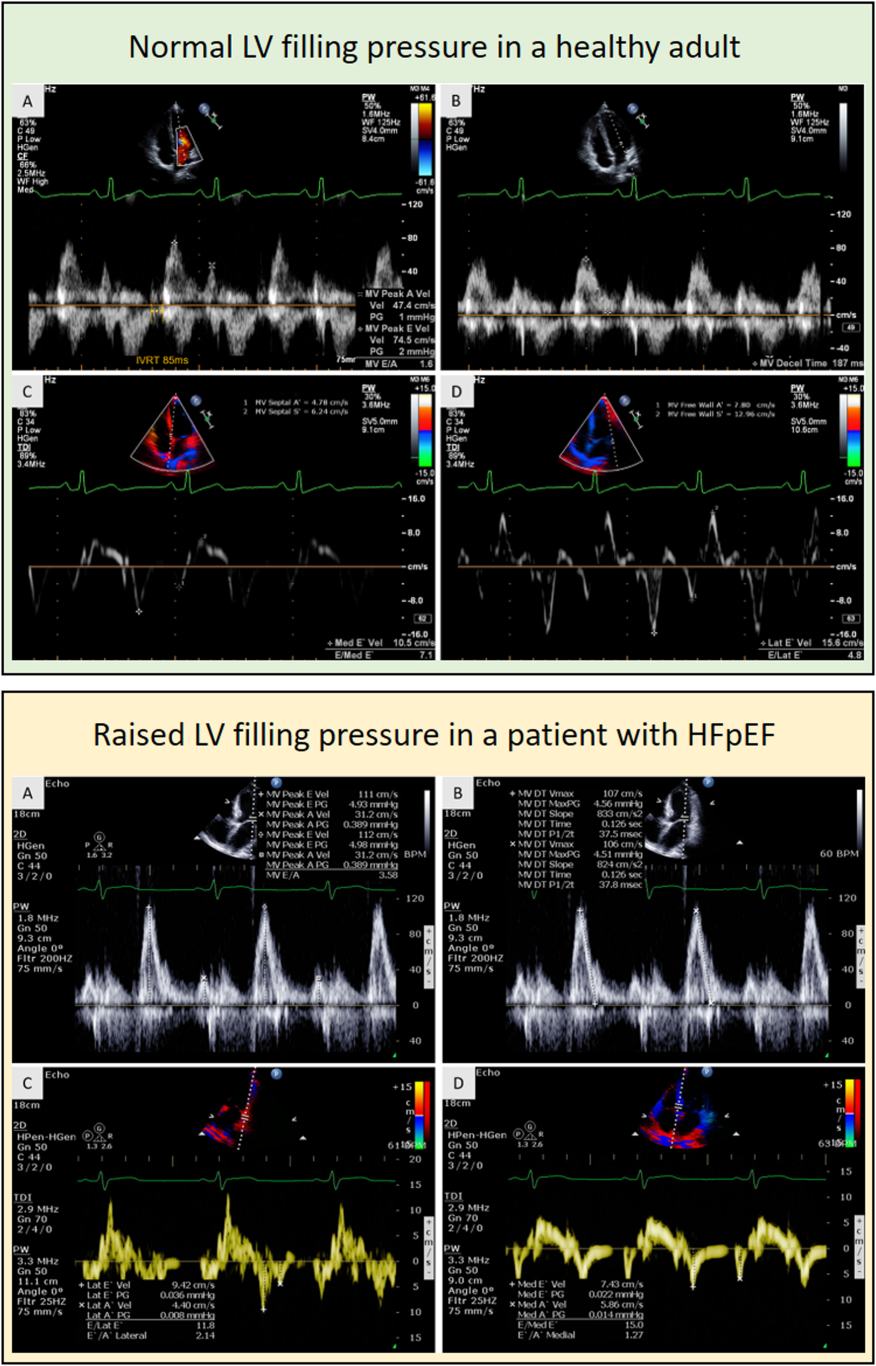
Case examples of Doppler‐based methods for left ventricular filling pressure (LVFP) assessment. The first case (green panel) is a healthy 20‐year‐old male; the second case (yellow panel) is a 60‐year‐old male patient admitted with heart failure (HF). In the healthy adult case, the E/e′ is <8 and suggests normal LVFP vs. in the patient, it is >12 and suggests raised LVFP.
